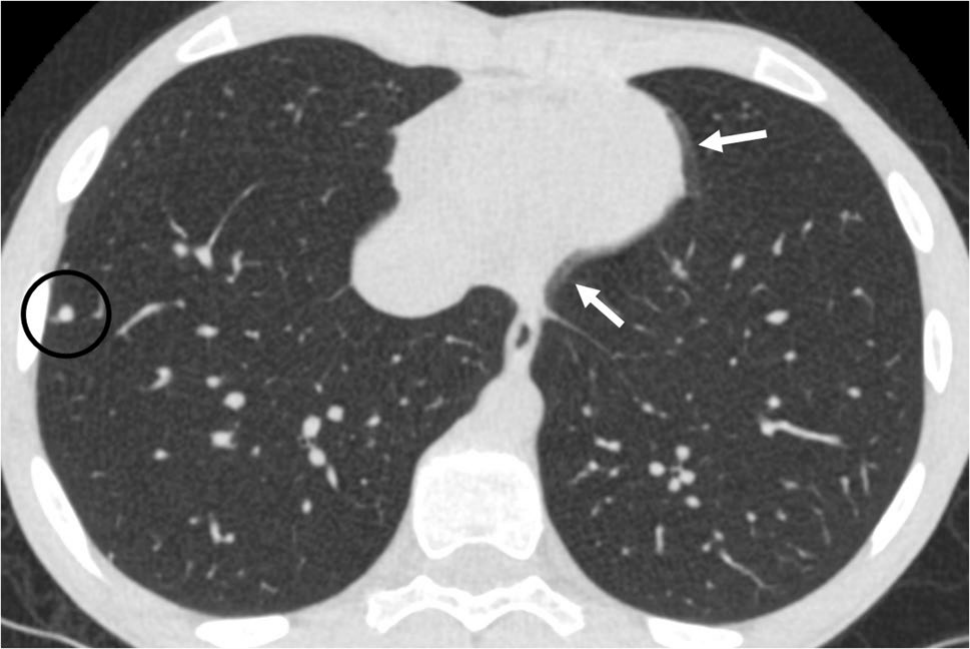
Representative axial lung computed tomography image of a 13-year-old boy, demonstrating cardiac pulsation artefacts (*white arrows*) and a lung metastasis (*black circle*). The examination was performed with a dose-length product of 5.73 mGy·cm, a volume computed tomography dose index of 0.22 mGy, a size-specific dose estimate of 0.36 mGy, and an effective dose of 0.22 mSv
